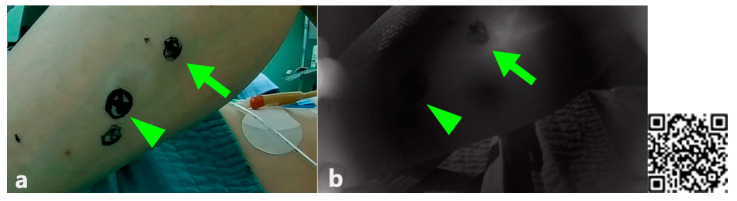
Different outcome in locating perforators between two devices(Doppler and ICG-NIRF prototype). (a) Locating perforators with Doppler, (b) locating perforators with a DIY ICG-NIR prototype (note that both arrow and arrowhead areas were positive on Doppler signal; however, only the site with the arrow was fluorescent), (c) QR code for ICG, free flap, and post-operative flap monitoring.
